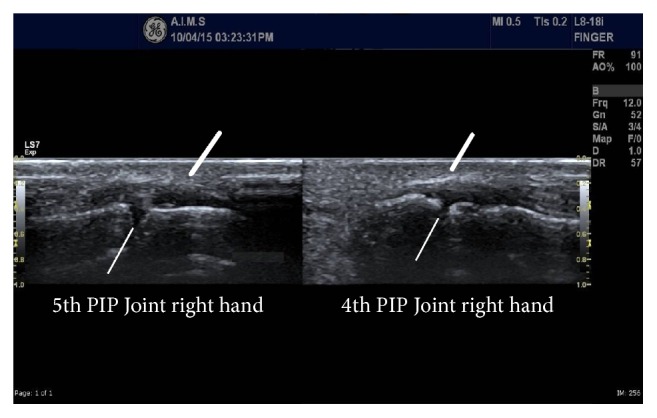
B-mode high resolution ultrasonography image (longitudinal section) of proximal interphalangeal joints showing diffuse dermal and subcutaneous oedema (thick lines) and normal joint space with no evidence of synovitis (thin lines).
